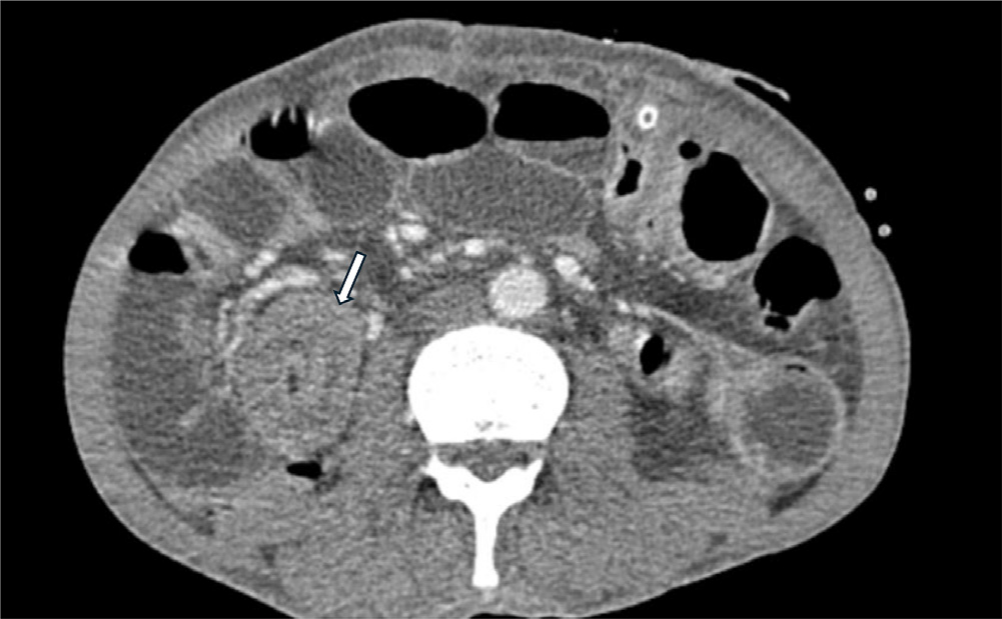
Axial abdominal CT scan showing the classic ``target sign'' (white arrow) consistent with intestinal intussusception around a feeding jejunostomy tube.
